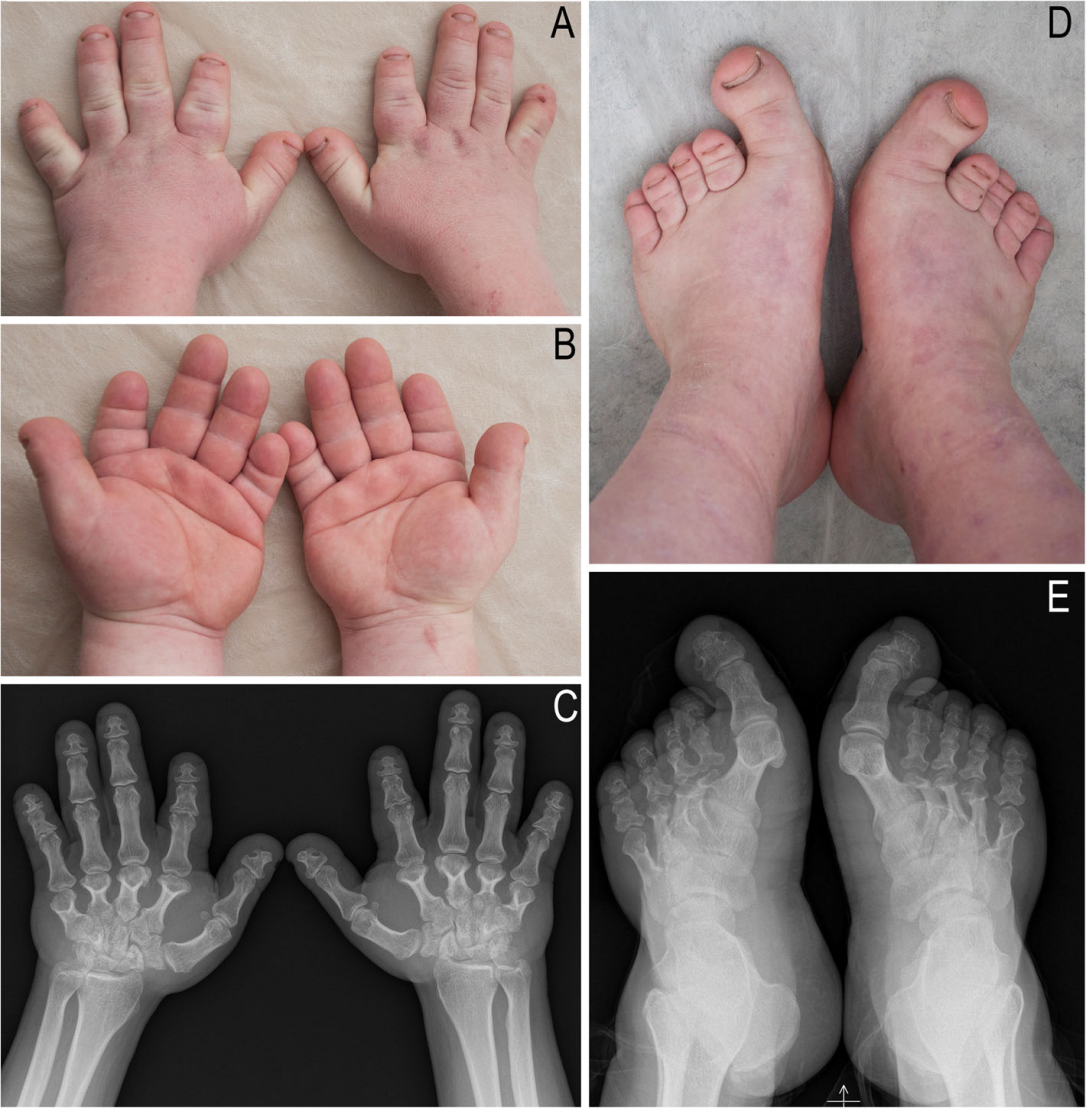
The photographs and X-ray pictures of hands and feet of the patient. The photographs of hands (a-b) and feet (d) show brachydactyly of both hands, a single palmar crease, short rectangular nails, brachydactyly of toes II–V of both feet, short rectangular toenails, and relative hyperplasia of the halluces. X-ray pictures (c, e) show the short broad metacarpals and phalanges
